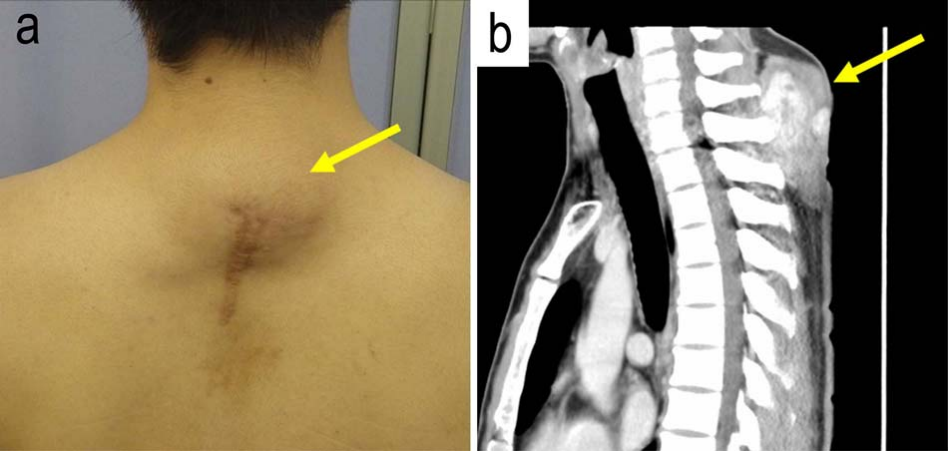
The gross and magnetic resonance imaging (MRI) finding of the tumor; a subcutaneous tumor beneath operation scar was located on the posterior neck of the patient (a); MRI study visualized a mass in the subcutis of the neck (b).
